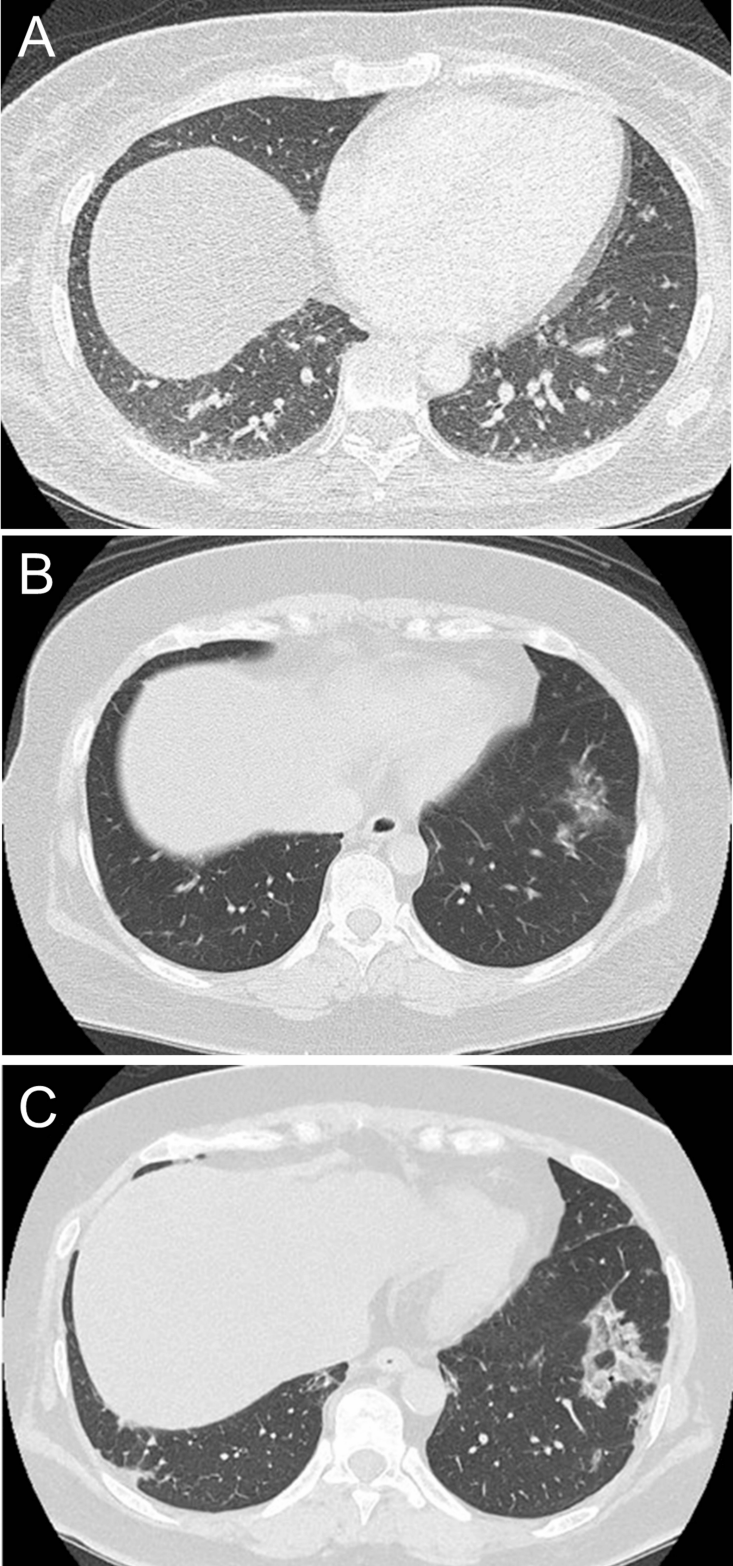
Chest computed tomography of the first case (A) Ground-glass opacities at the time of diagnosis in bilateral dorsal lung fields. (B) Ground-glass opacity only in the base of the left lung at the first recurrence. (C) Development of a new consolidation in the lower part of the left lung at the second recurrence.
